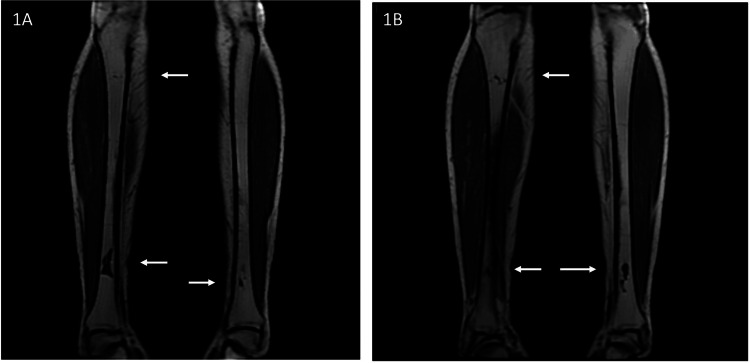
Two consecutive coronal MRI images of the legs on T1-weighted image revealing serpiginous areas (white arrows) of low T1 signal intensity in the medullary cavity of proximal and distal tibial diaphyses bilaterally, suggestive of medullary infarcts
